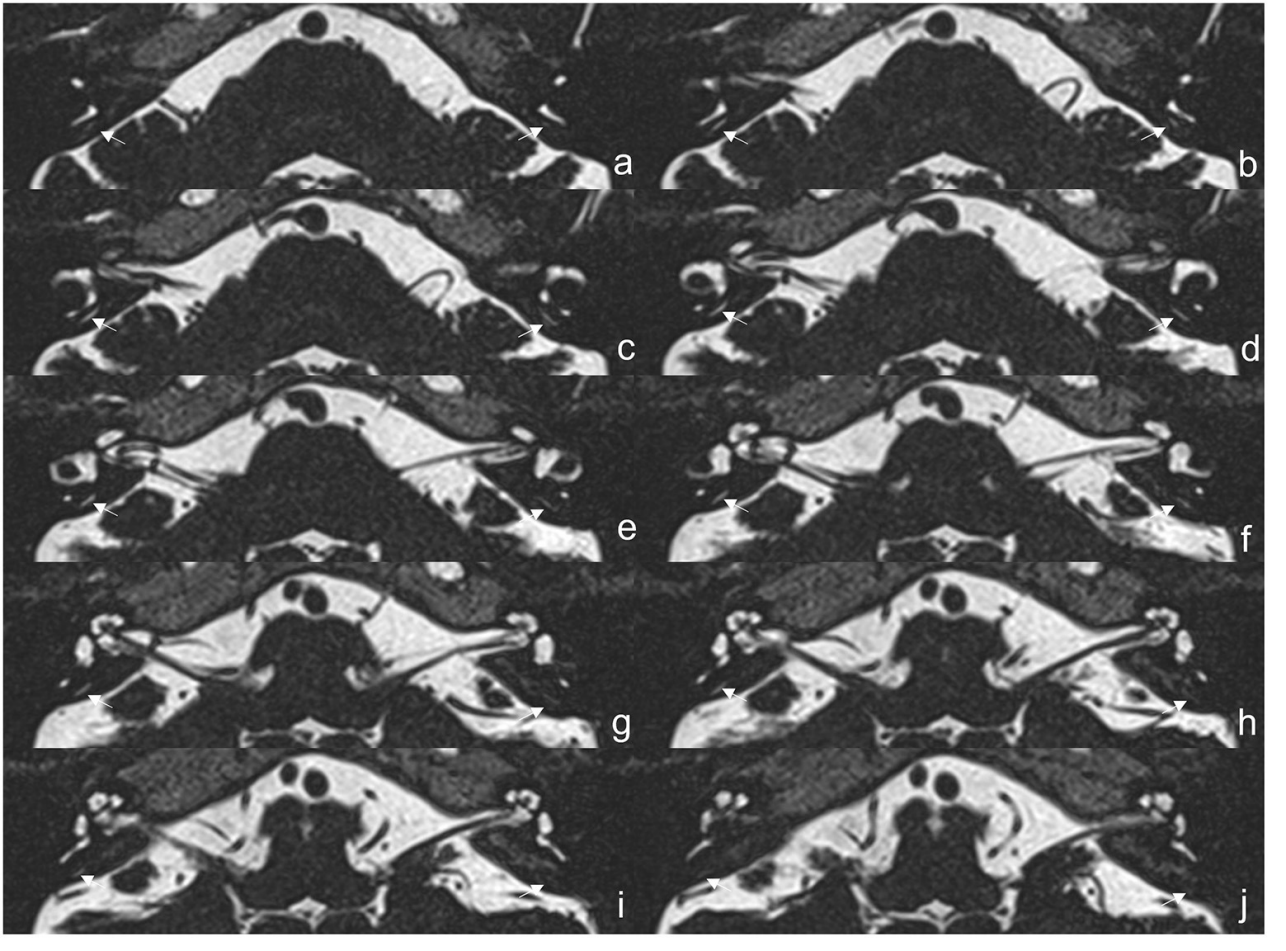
The 3D-SPACE MRI images of a 57-year-old female with vestibular migraine (VM). (a–j) Axial, high-resolution, and T2-weighted MRI scan showing visualization of the vestibular aqueduct on both sides. *3D-SPACE*, three-dimensional sampling perfection with application optimized contrasts using different flip angle evolutions, *VM*, vestibular migraine.
